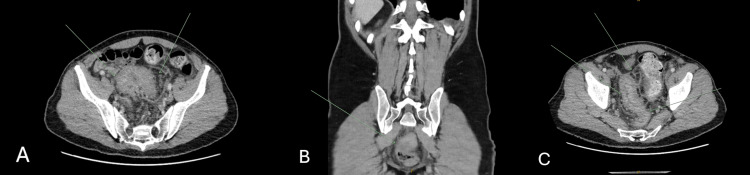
CT slices demonstrating rectosigmoid colitis on axial (A,C) and coronal (B) views
